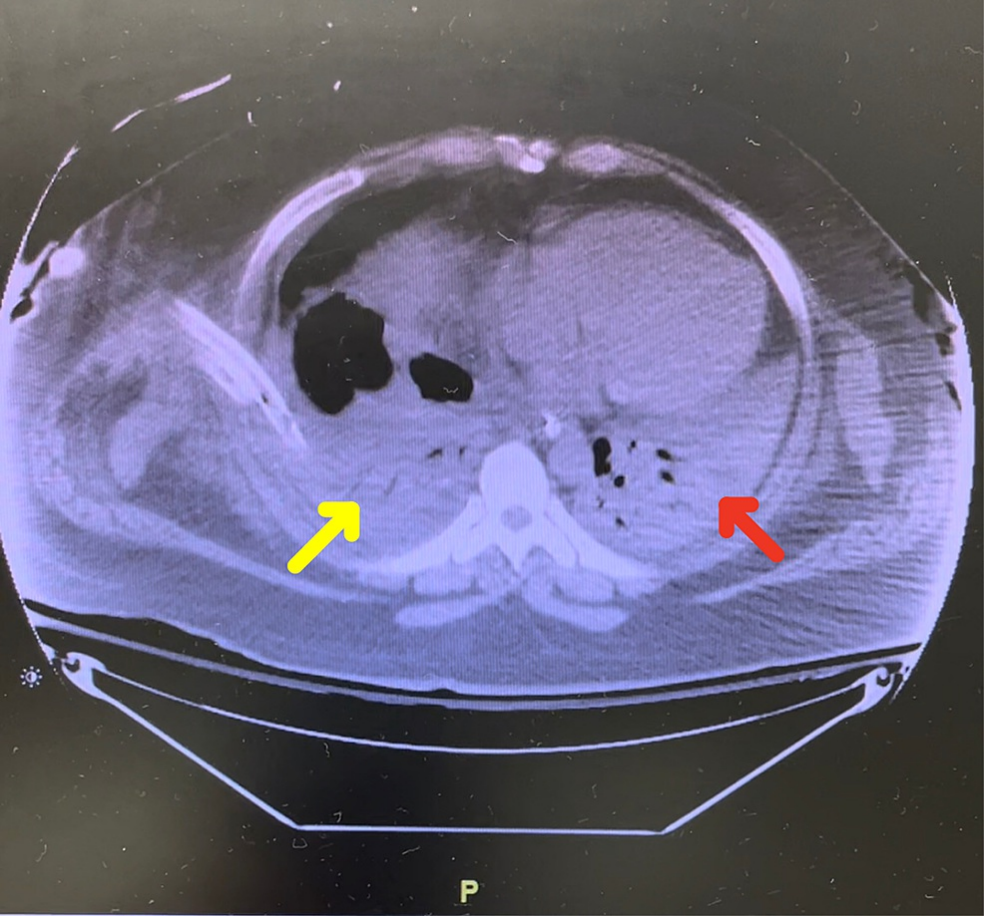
CT of the chest from day 8/33 of hospitalization showing complete atelectatic collapse of the left lung (red arrow) and right lung and a loculated right basilar hydropneumothorax (yellow arrow)
